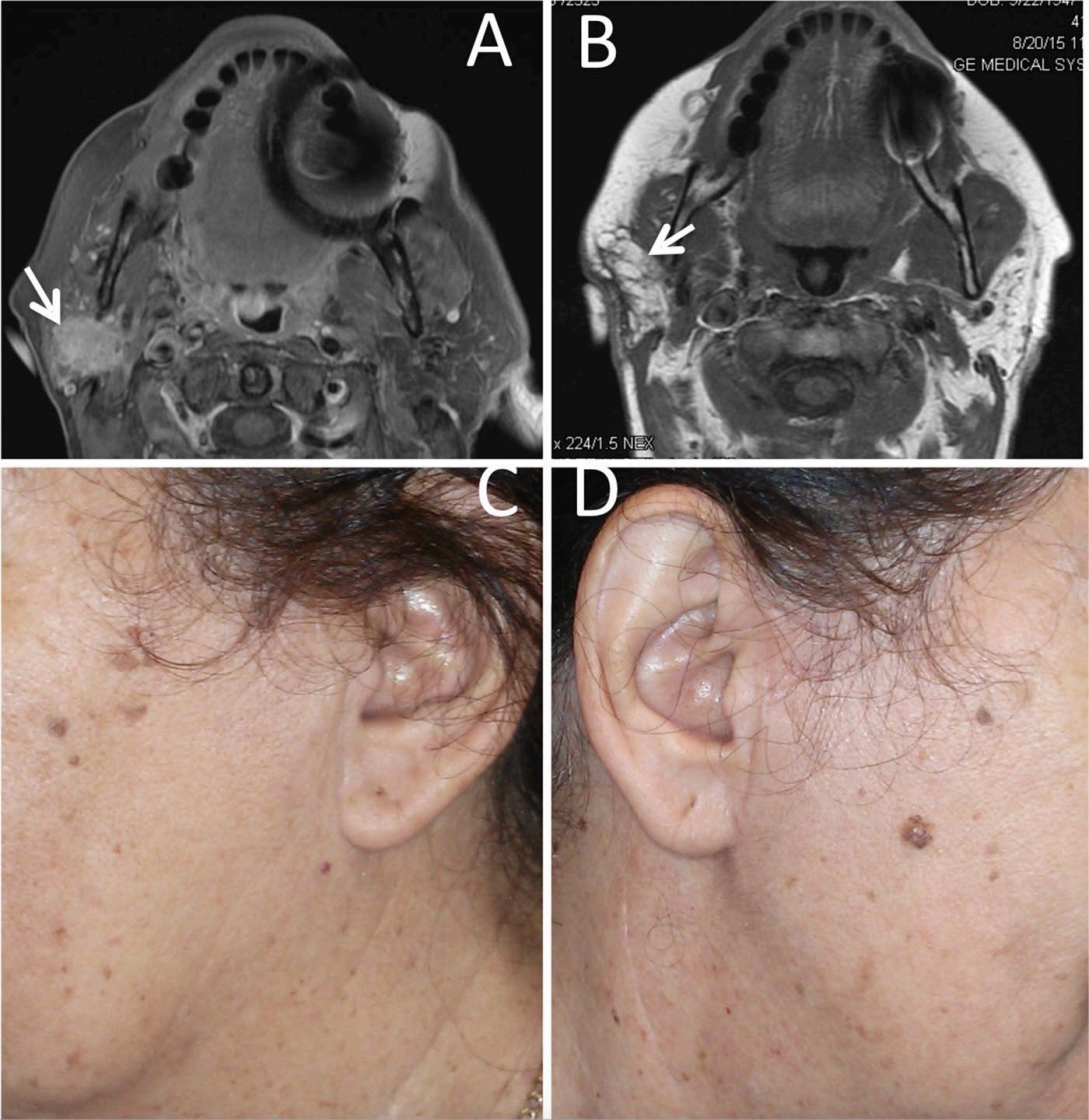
Dermal-fat-fascia graft at 1.5 years following reconstruction for a right lateral parotidectomy defect for a mammary analog secretory carcinoma. (A) MRI imaging showing the carcinoma prior to parotidectomy. (B) MRI imaging showing the graft after parotidectomy. (C) Facial contour of the non-operated side. (D) Facial contour of the operated side.
